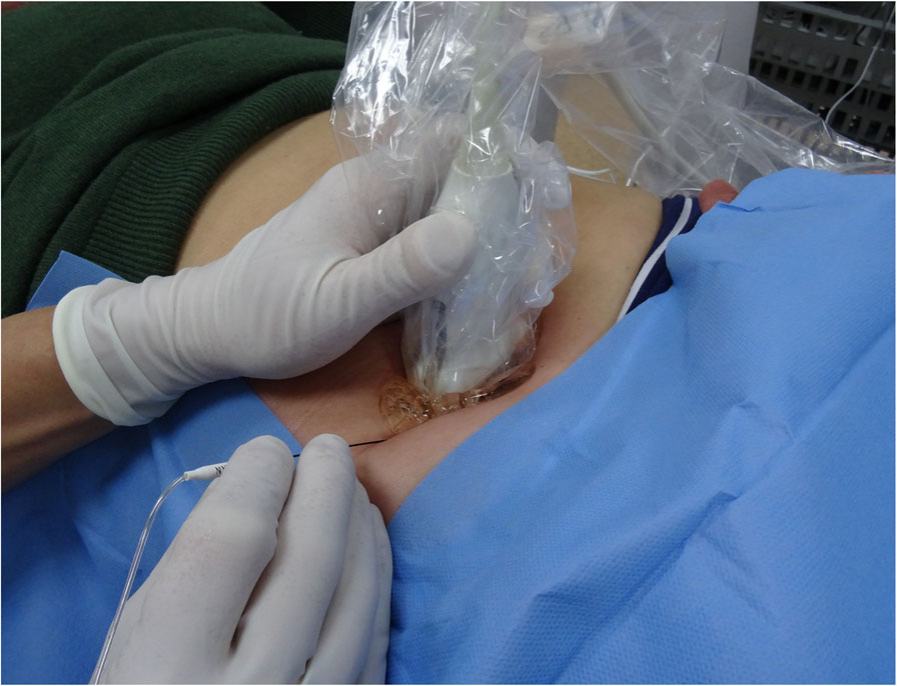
Ultrasound-guided placing of the pulsed radiofrequency (PRF) cannula at the tender point
